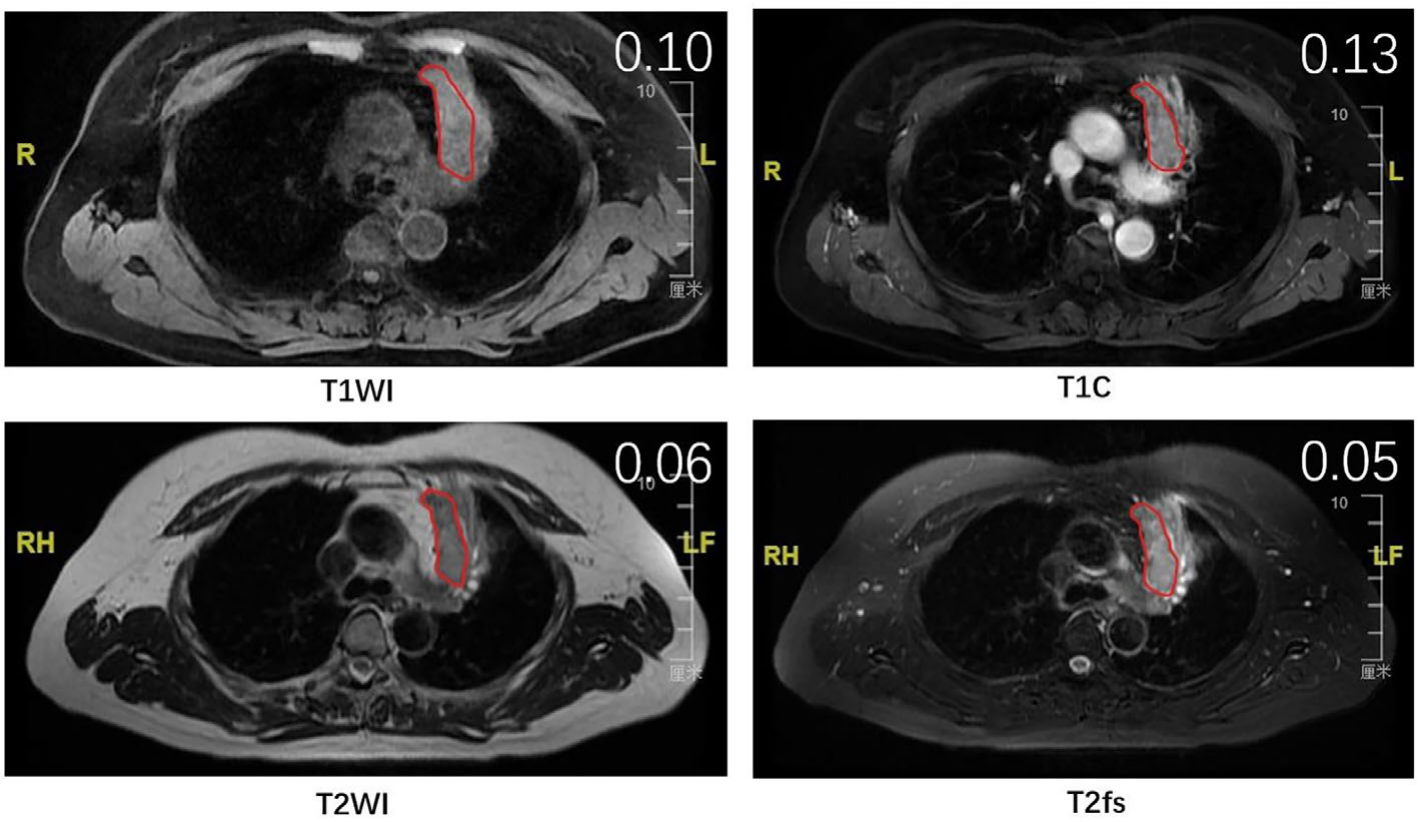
The MR images of a patient with stage T4 that was falsely predicted as stage T2 by the model. Red lines delineate the ROI, which was delineated on the largest cross-sectional slice of primary lung tumor. The SD/mean value was shown on the right upper top of each image.
